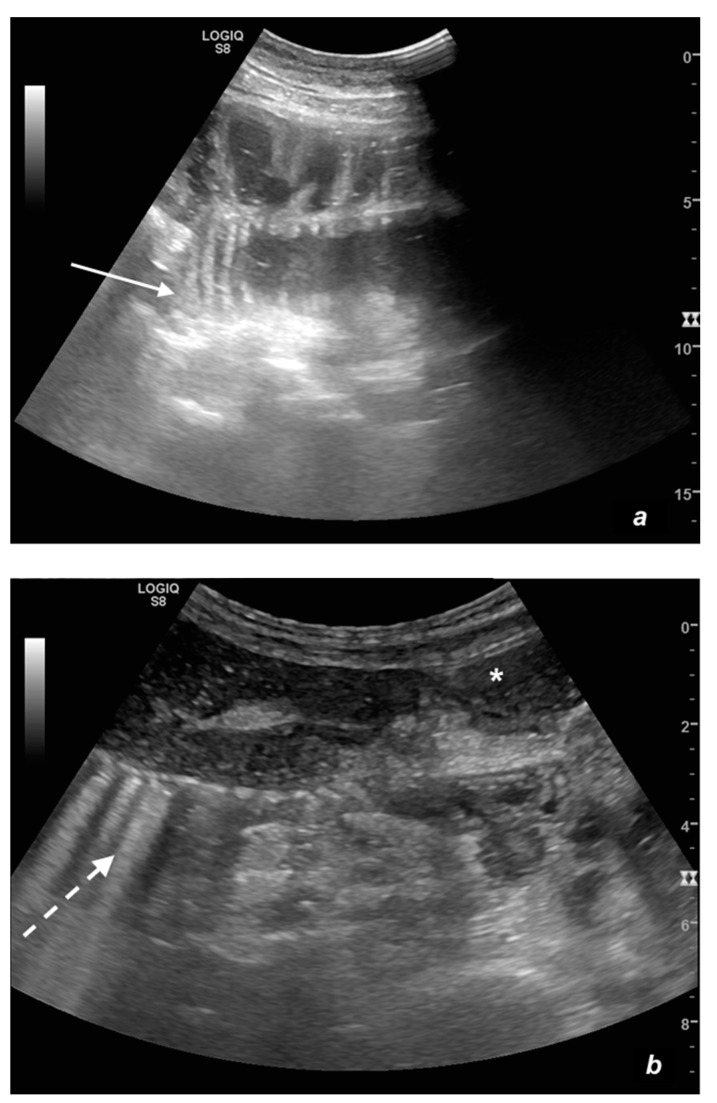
(a,b). A simple SBO. Ultrasound images show fluid-filled, dilated small bowel loops (a,b) with hyper-kinesis and hyper-representation of valvulae conniventes (white arrow) (a); tail comet artifacts are visible due to air-fluid levels (dashed arrow) (b) and groups of bowel loops with severe differences in diameter (‘bowel jump diameter’) are evident more superficially (*) (b). No free fluid was detected in the abdominal cavity or between bowel loops.
